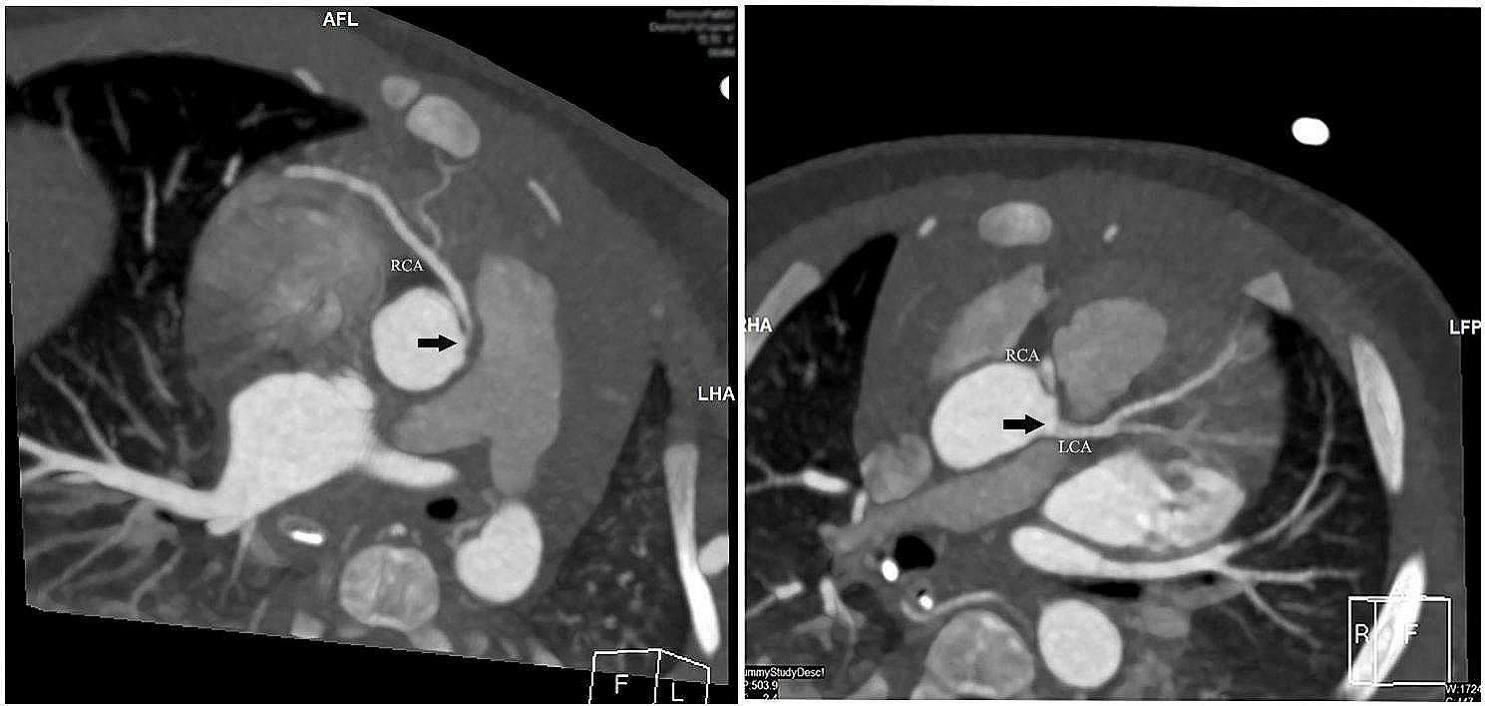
CT coronary angiography shows the anomalous origin of the RCA from the left sinus of Valsalva (Black arrow). RCA, Right coronary artery; LCA, Left coronary artery
